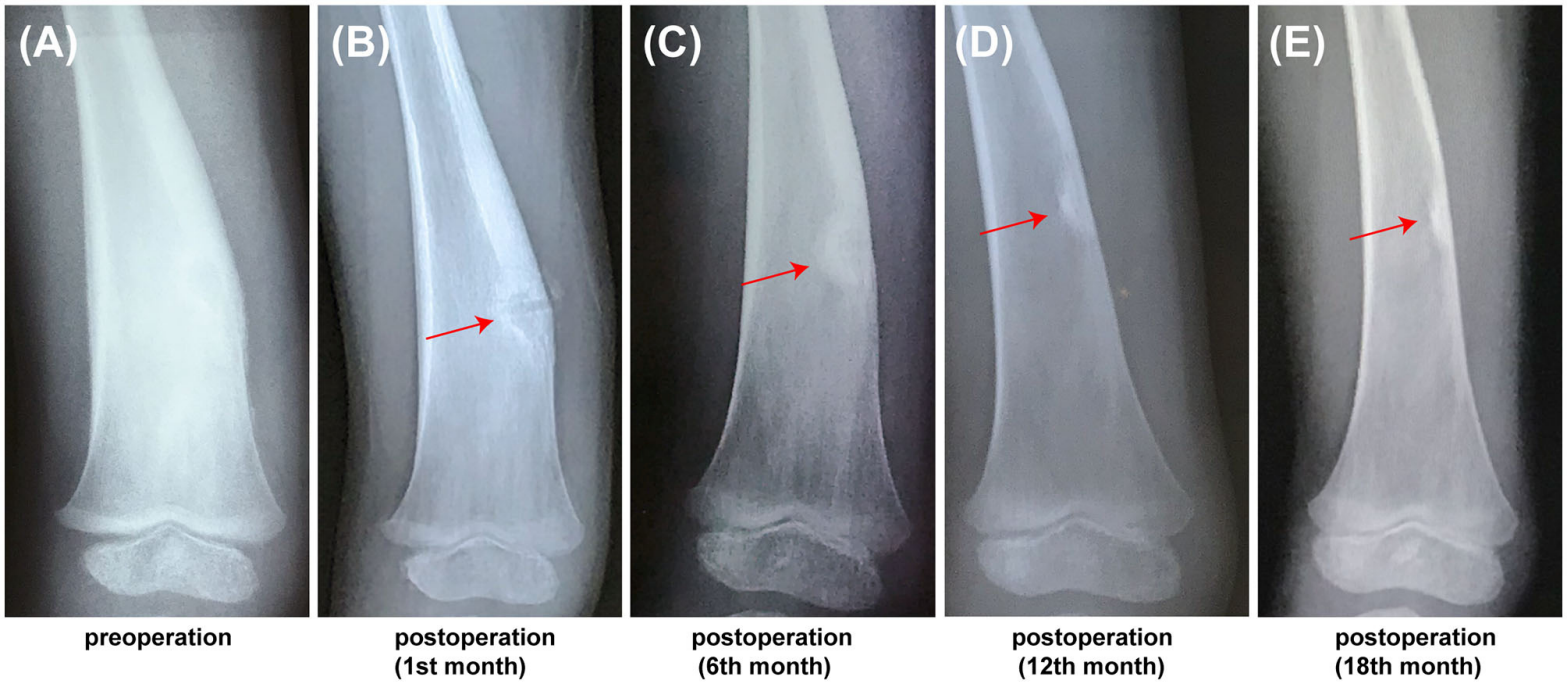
The follow-up radiographs of a 3-year-old child confirmed with OO using the robot-assisted surgery. (A) Pre-operative X-ray image showed showing the thickening of cortex. (B) PostoperativePost-operative X-ray image at 1st month, (C) Post-operative X-ray image at 6th month. (D) Post-operative X-ray image at 12th month. (E) Post-operative X-ray image at 18th month (red arrow, the nidus).
